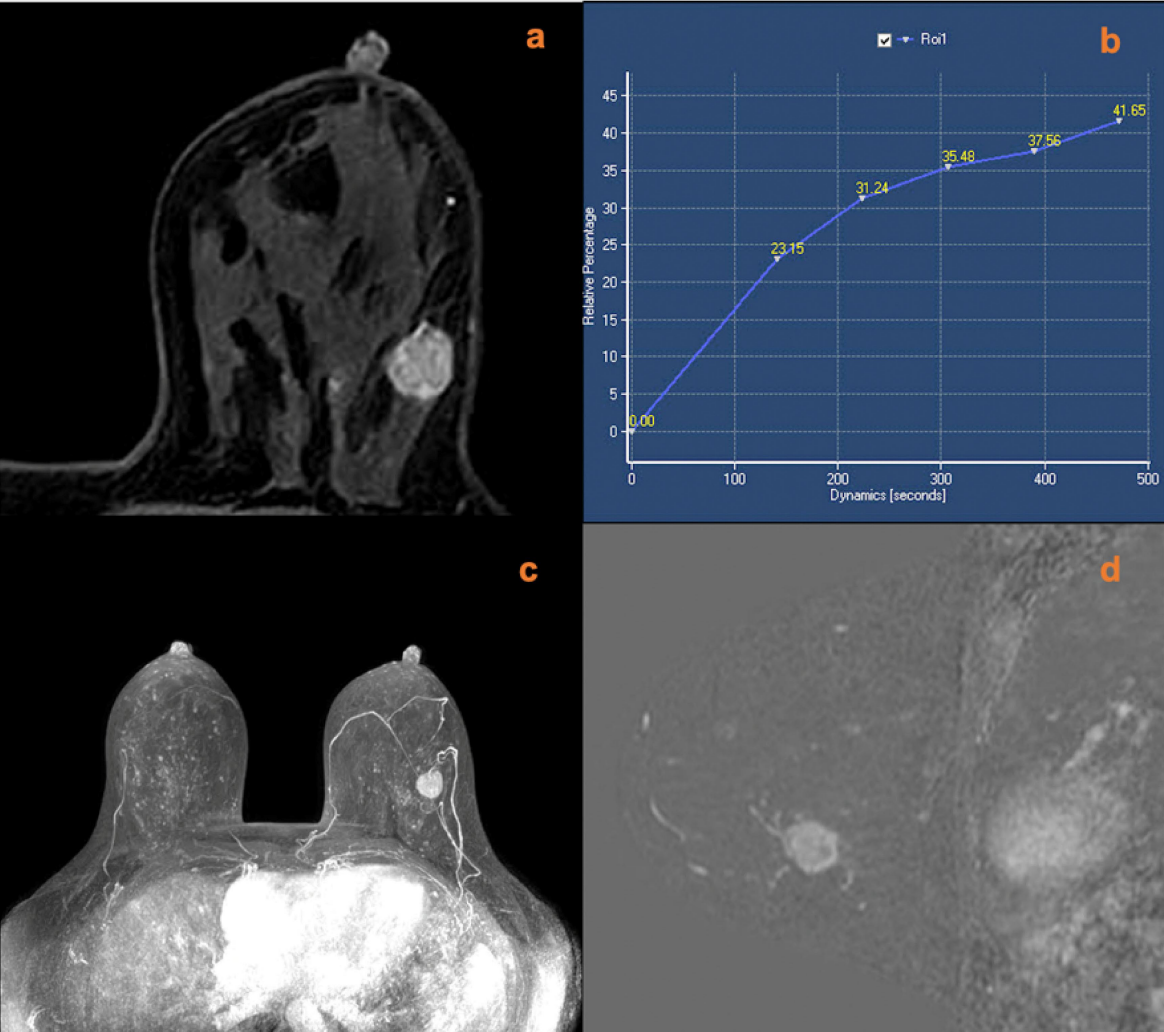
A 51-year-old female was newly identified with a left breast mass lesion for 10 days. MRI (A, contrast-enhanced axial; B, time signal intensity curve; C, maximum intensity projections image; D, subtraction image) shows a left breast mass lesion with an irregular margin and persistent enhancement. The lesion also increased the ipsilateral breast vascularity and the positive AVS sign. The KS of the lesions was 3, while KS^*^ was 6. Postoperative pathology confirmed that the lesion was an invasive ductal carcinoma.
